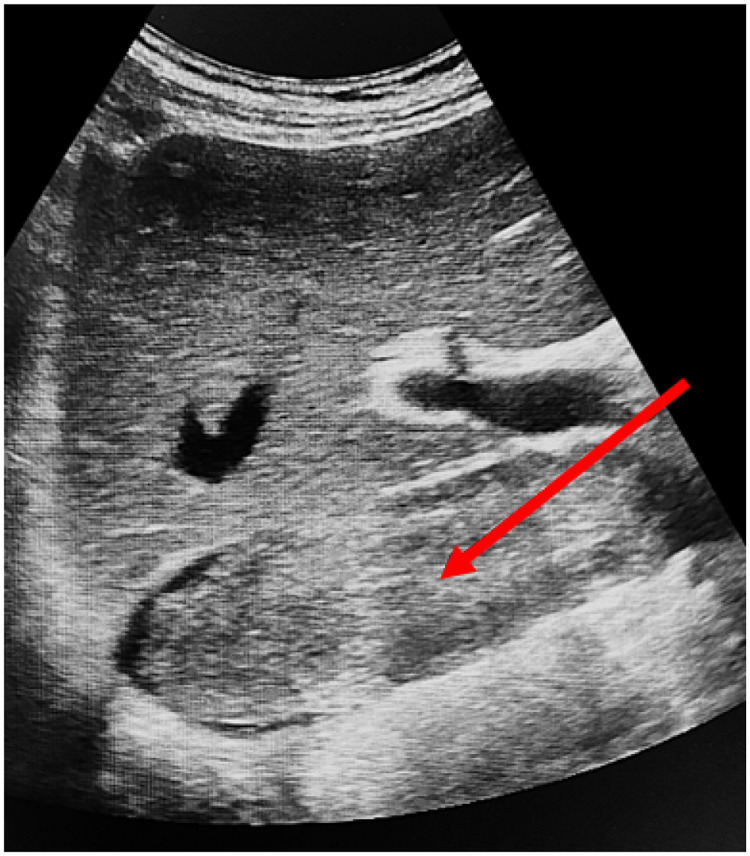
Abdominal ultrasound. Tumor thrombus in the retrohepatic segment of the IVC (red arrow).
